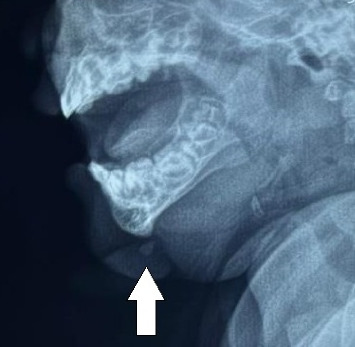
Plain Lateral Radiograph of the face showing a well-defined soft tissue opacity extending from the mental protuberance of the mandible anteroinferiorly with a focus of radiodensity similar to the tongue and other soft tissues within.
